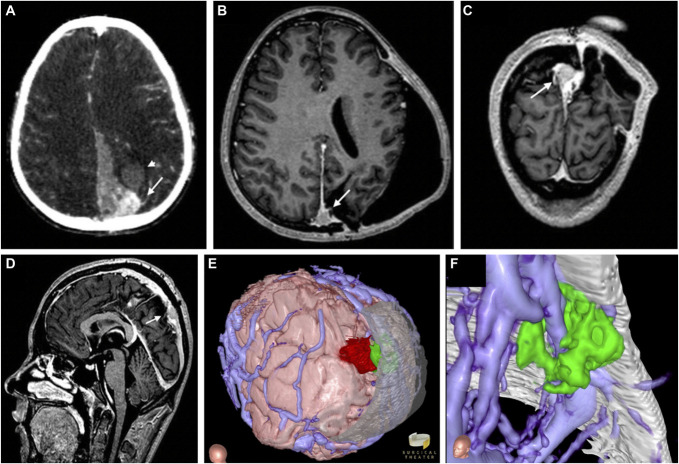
Hemorrhagic parietal synovial sarcoma. A, Axial noncontrast computed tomography shows a hyperdense lesion (arrow), intraparenchymal hematoma (arrowhead), interhemispheric hemorrhage, and 6 mm of midline shift. Postoperative B, Axial, C, Coronal, and D, Sagittal T1-weighted MR images show a residual contrast-enhancing lesion (arrows) along the superior sagittal sinus. E, Three-dimensional reconstruction of blended computed tomography and magnetic resonance imaging recreates the hemorrhagic tumor resected during the patient's first surgery (crimson) and residual tumor underlying the craniotomy edge (green). F, A “bottom-up,” intracranial vantage point reveals margins of the tumor underside of the superior sagittal sinus extending contralaterally.
